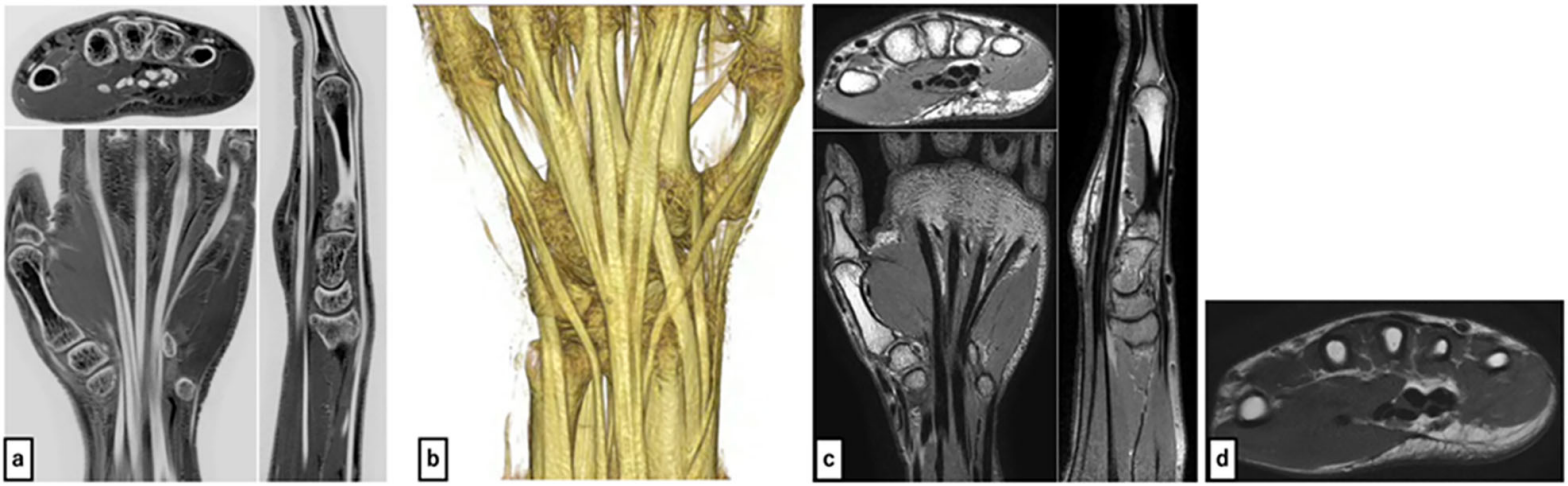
a FRACTURE sequence (axial, coronal, and sagittal reformation) shows tendons as distinct high signal intensity similar to cortical bone, with clear contrast against surrounding musculature. b Volume-rendered image from the FRACTURE sequence depicts the tendon course alongside skeletal structures, facilitating easy assessment of an individual patient’s tendon conditions. c PD-VISTA sequence (axial, coronal, and sagittal reformation) shows tendons as low signal intensity structures and are relatively clearly visualized. d Axial T2-weighted imaging sequence depicts tendons as low signal intensity structures with relatively clear visualization
